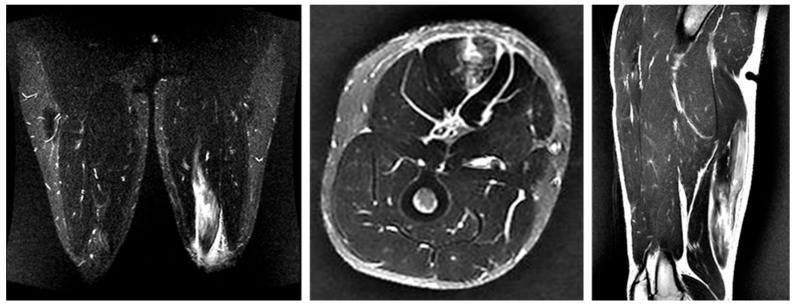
Case 6 MRI images: ST distal MTJ injury, Type III. (A) (Coronal image): A haemorrhagic lesion is observed at the distal MTJ. (B) (Axial image): prone position, A lesion of the intramuscular tendon of the ST distal tendon is observed. (C) (Sagittal image): A lesion is observed at the distal MTJ of the ST.
